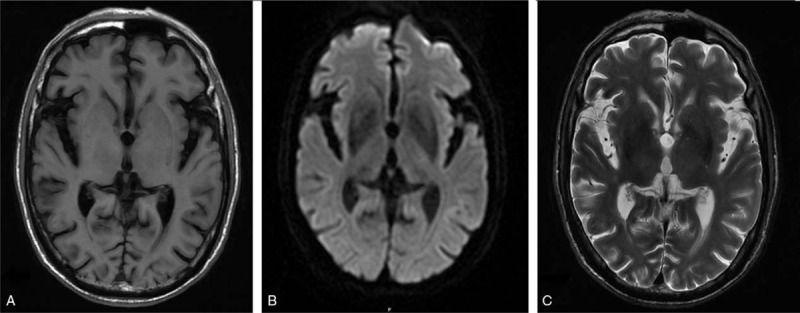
Brain magnetic resonance imaging showed no infarction or hyperintensity lesion in the right basal ganglion (A) T1-weighted brain magnetic resonance imaging (B) diffusion-weighted imaging (C) T2-weighted brain magnetic resonance imaging.
